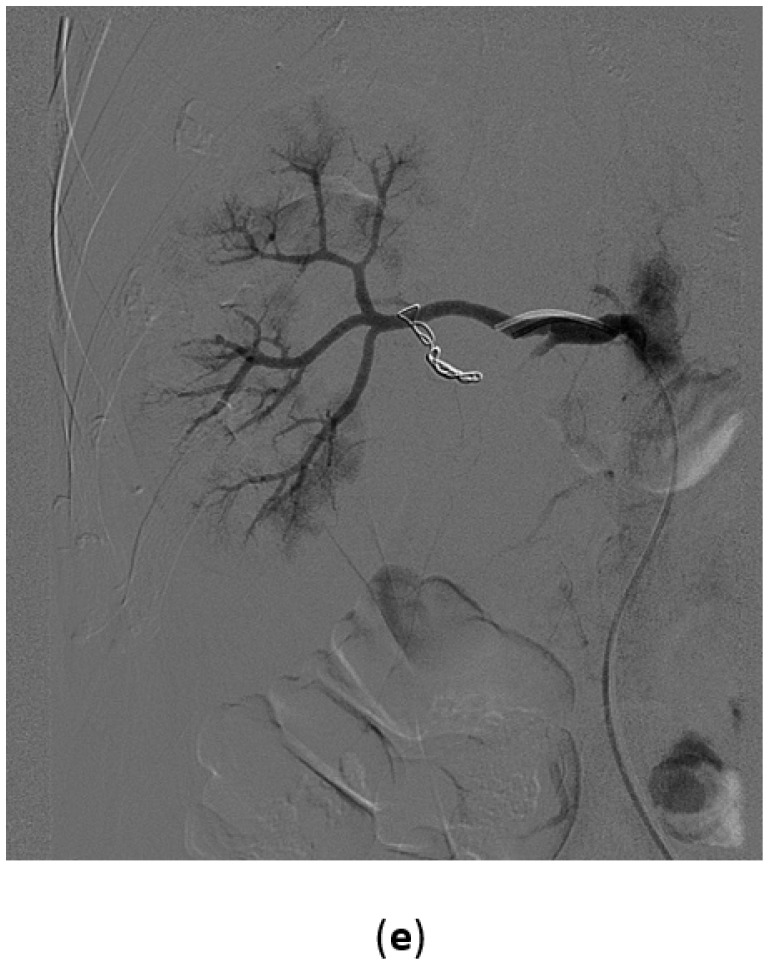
Angiomyolipoma. 54-year-old female with sudden onset of right flank pain. (a) Unenhanced CT: the right kidney is dislodged anteriorly by a perirenal hematoma with a mixed pattern consisting of fat attenuation density and high attenuation density due to acute bleeding; (b,c) Contrast enhanced CT: AML shows a “jet” sign due to active extravasation inside the mass; (d) Selective angiogram of the feeding artery confirms the typical aspects of AML: rich vascularization with aneurysmatic aspects; (e) Complete devascularization of the AML after embolization with particles and microcoils.
